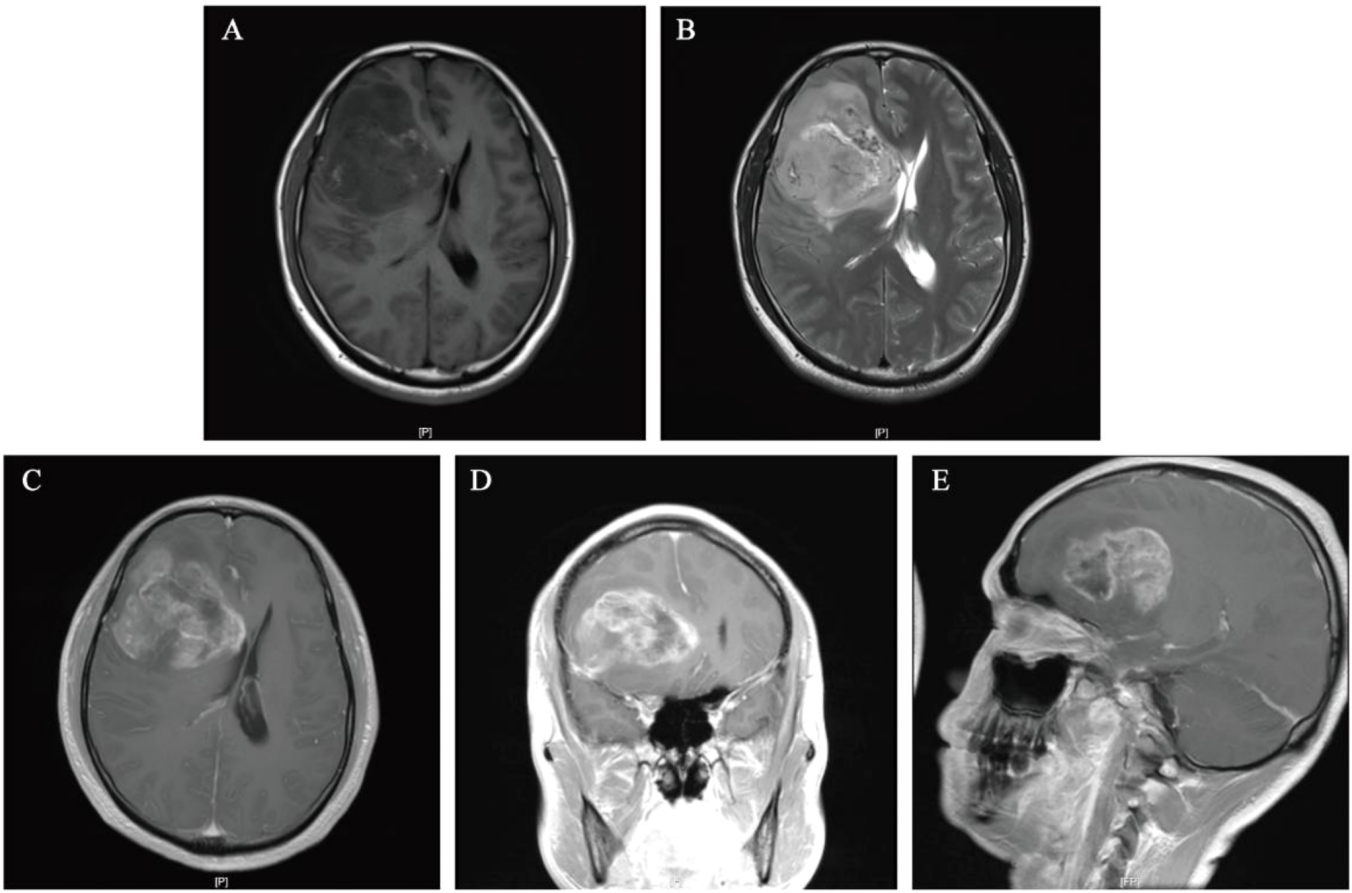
Preoperative MRI images of head. (A, B) T1-weighted sequences and T2-weighted sequences. (C-E) The lesion exhibits pronounced enhancement at its margins and within its solid components on T1-weighted contrast-enhanced axial, coronal, and parasagittal MRI.
